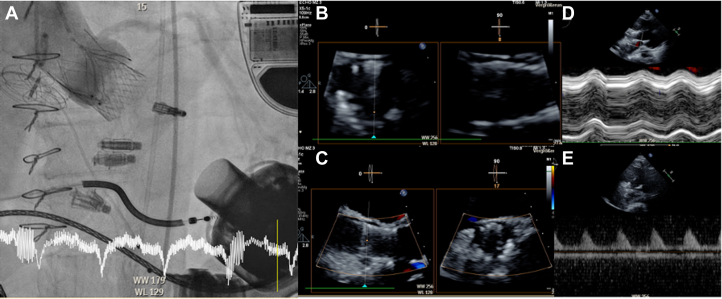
Case 1: Postimplantation Imaging (A) Postimplantation aortography confirms correct valve positioning. (B-E) Multimodal echocardiographic assessment including (C) color Doppler, (D) M-mode, and (E) continuous-wave Doppler all demonstrate absent transvalvular flow, consistent with hemodynamic valve closure due to insufficient opening gradient.
